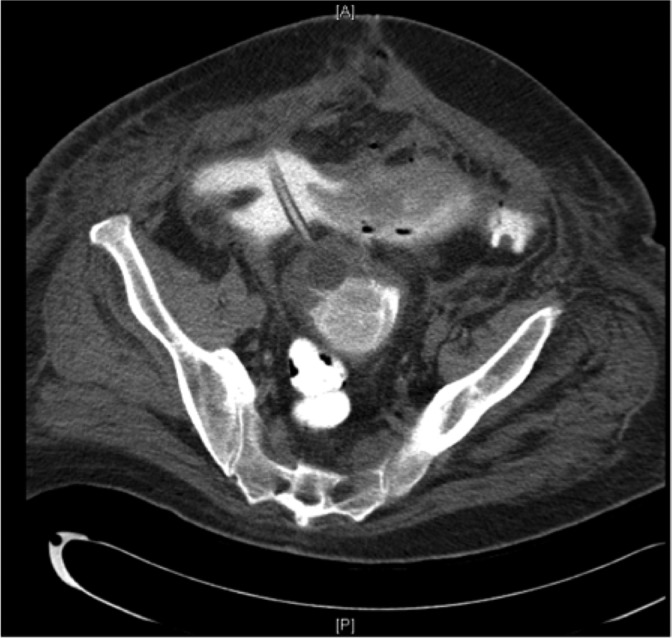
CT axial abdomen/pelvis with contrast. Extravasation of contrast from bladder. Large pelvic hematoma in close proximity to bladder. CT, computed tomography.
